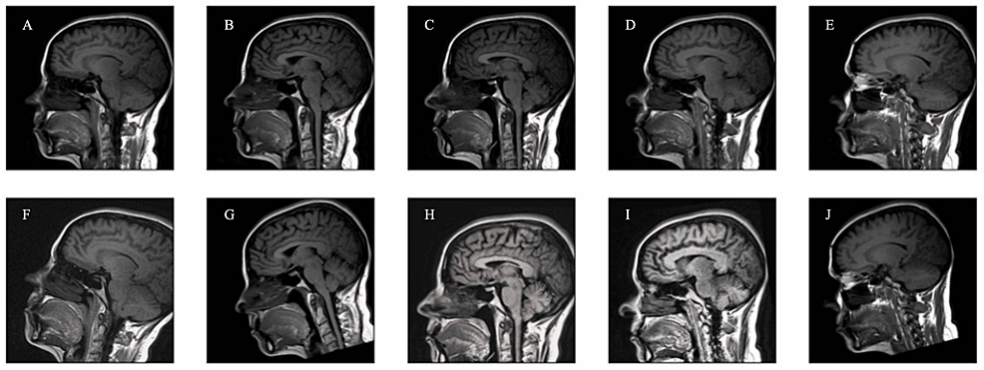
Examples of images following data augmentation in the dataset A) Original MRI slice. (B-J) Examples of multiple augmented versions that were created for each MRI slice. The augmentation included a range of techniques such as random shifts of 10%; scaling by 10%; rotation of ±20°; cropping, sharpening, and blurring; perspective transformations; addition of Gaussian noise; adjustments in brightness and hue saturation value levels; and gamma transformations. MRI, magnetic resonance imaging
